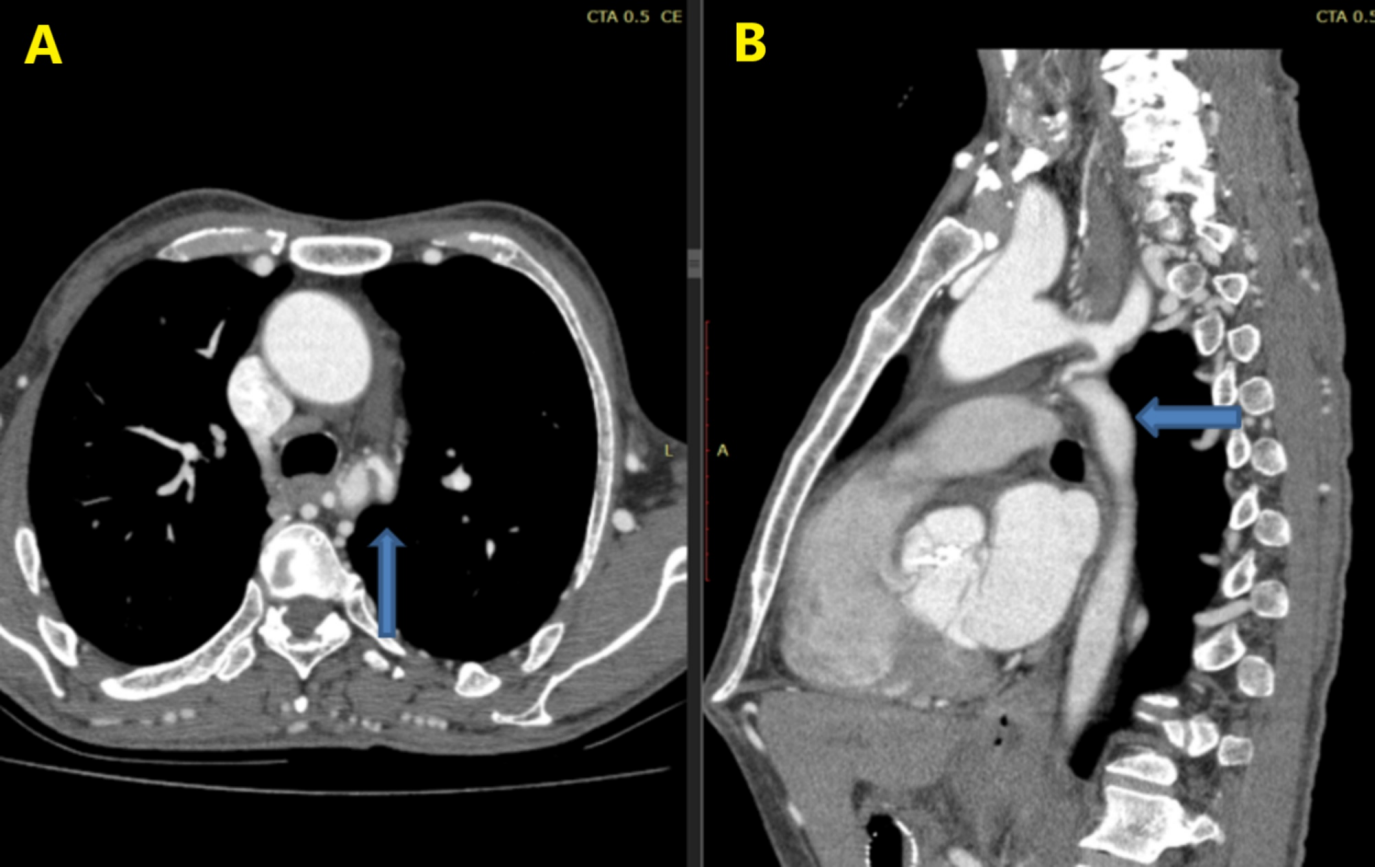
CT scan showing a narrowed segment of the aorta: (A) axial, (B) sagittal.
